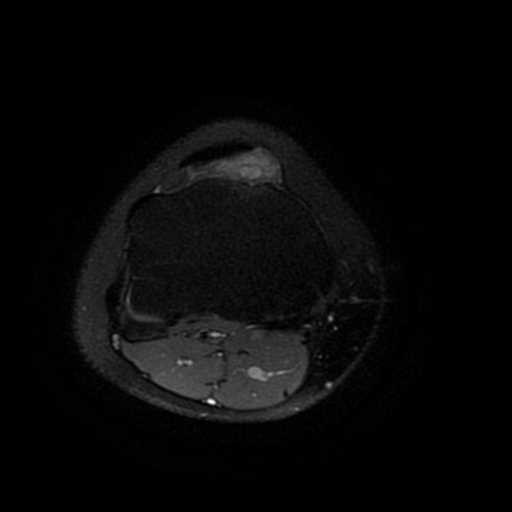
T2-weighted axial view of the knee displaying the lesion with a bright signal under the patellar tendon.
